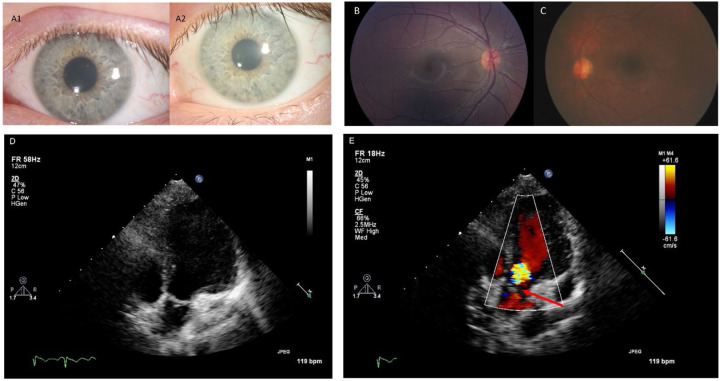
Selected ophthalmology and cardiology results. Panels A1 and A2: Corneal slit-lamp photographs from the same Juvenile GM1 patient showing slow progression of corneal clouding over a five-year period. Panel B: Left eye Topcon color fundus photograph from a Juvenile GM1 patient showing an essentially normal fundus with no evidence of macular or optic nerve head pathology. Panel C: Right eye RetCam fundus photography of a late-infantile GM1 patient showing normal macula and optic nerve with minimal retinal vascular tortuosity. Panels D and E: Two dimensional and color transthoracic echocardiographic imaging of the left ventricular outflow tract from a single Juvenile GM1 patient. The red arrow points to aortic valve leaflet thickening with associated aortic regurgitation. These images are representative of the abnormal thickening of the aortic valve leaflets with associated aortic regurgitation observed in three unrelated Juvenile GM1 patients (23% of those who received ECHO; an additional five participants had aortic and/or mitral valve leaflets that were qualitatively thickened for age but without regurgitation or stenosis).
